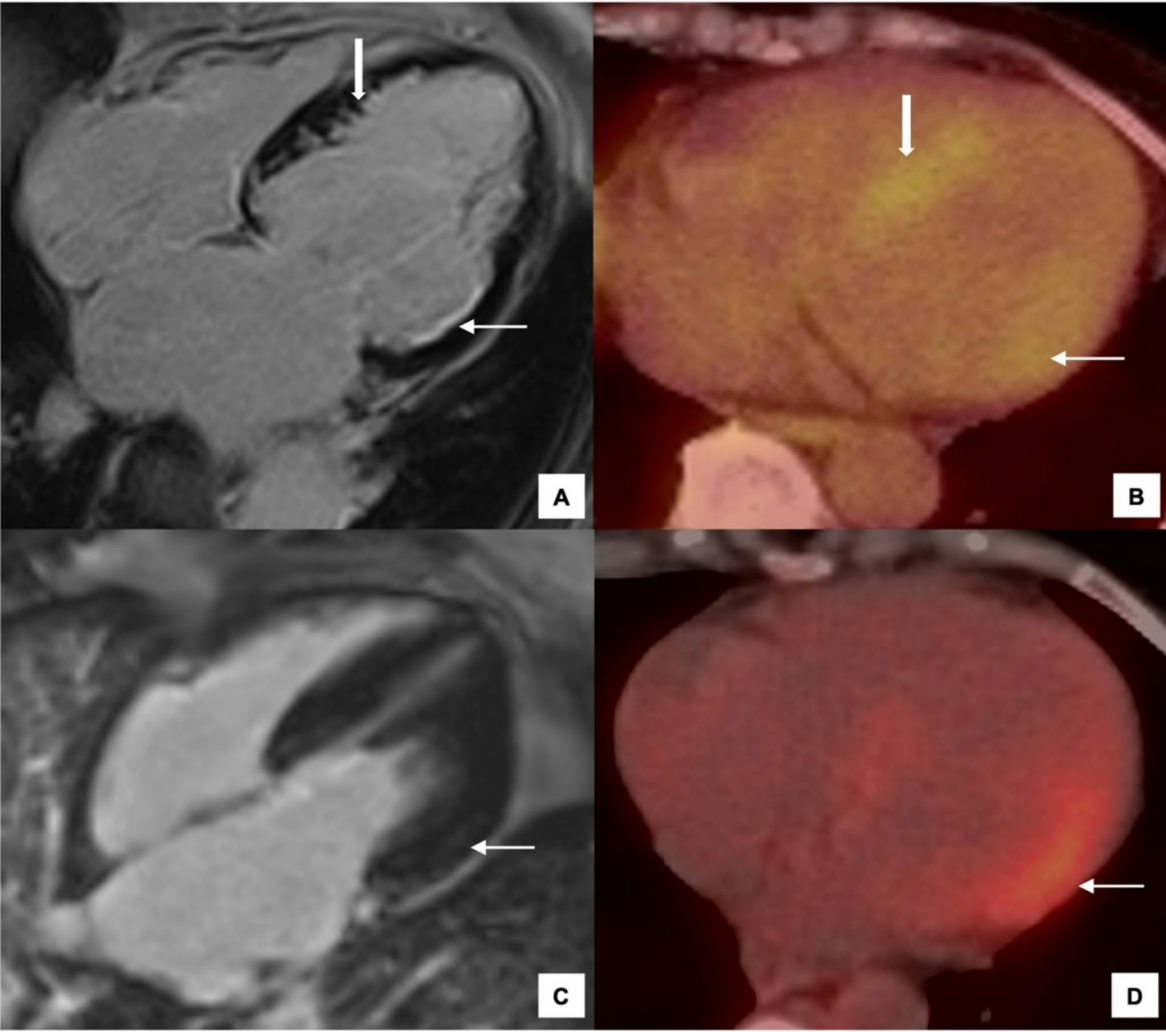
Use of FDG-PET imaging in EGPA. A-B: Example of active inflammation & scarring on PET. A: 4 chamber cardiac MRI shows subendocardial LGE in the basal lateral wall (*thin arrow*) and along the septum (*block arrow*). B: The corresponding FDG PET-CT demonstrates increased metabolic activity in the septum (*block arrow*) in keeping with active inflammation while there is only subtle increase in FDG uptake along the basal lateral wall (*thin arrow*) suggestive of established myocardial scarring. C-D: Increased sensitivity of PET to pick up active inflammation. C: 4 chamber cardiac MRI shows subtle LGE (*arrow*) in the basal lateral wall. D: FDG-PET-CT shows intense increased metabolic activity (*arrow*) in the basal lateral wall. Abbreviations: CT, Computed tomography; FDG, Fluoro-2-deoxyglucose; LGE, Late gadolinium enhancement; PET, positron emission tomography
